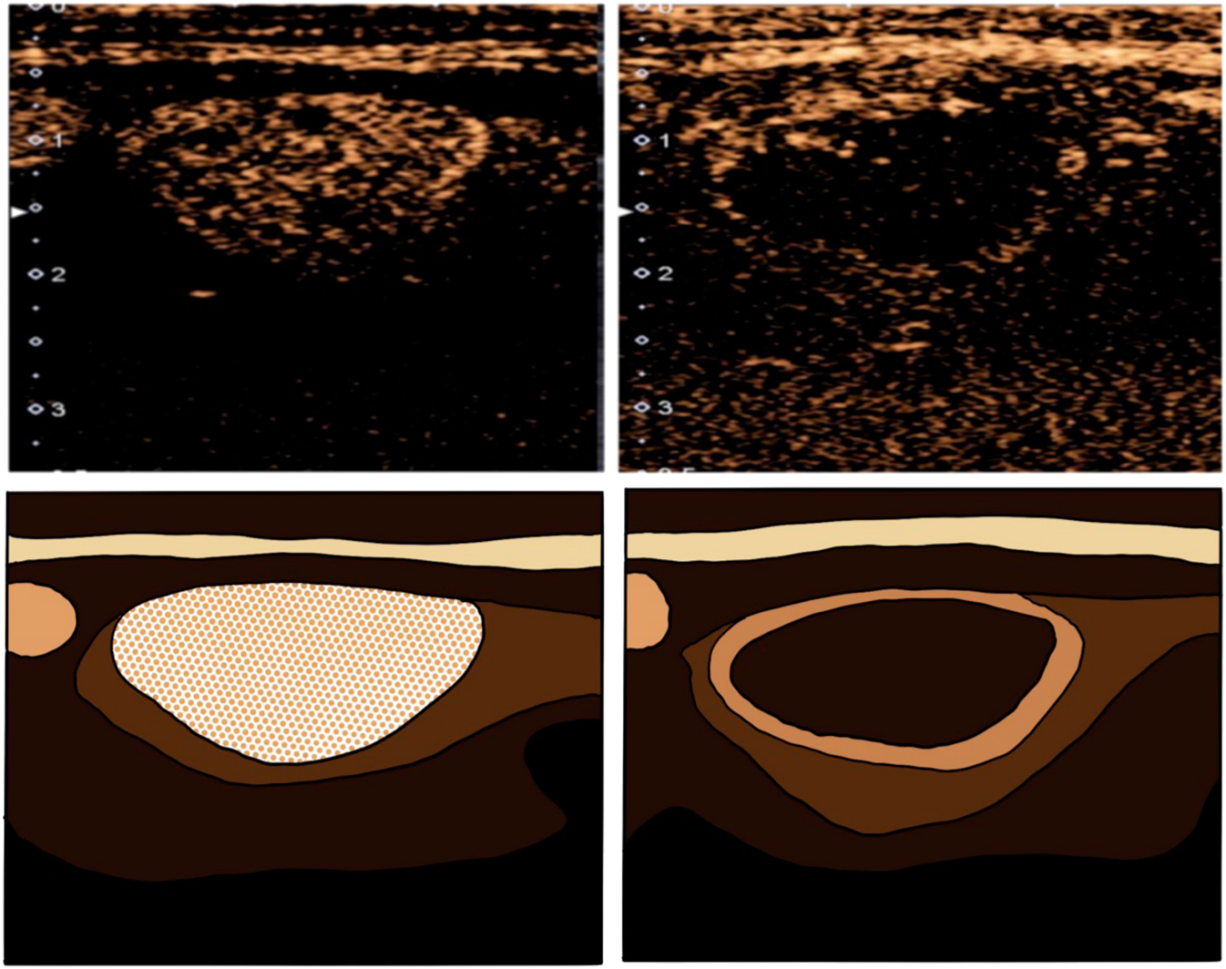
Use of ultrasound contrast before and immediately after ablation ensures treatment of at least 80% of the nodule.
